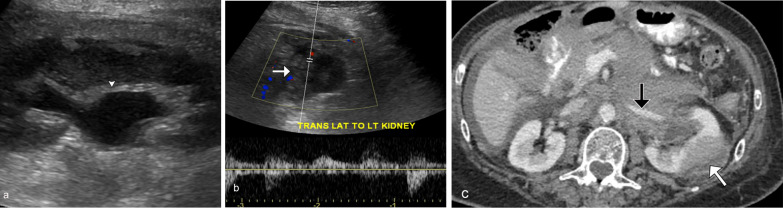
Direct extension from retroperitoneal adenopathy of renal lymphoma.* Grayscale ultrasound image (a) demonstrates mild hydronephrosis (arrowhead) of the left kidney. Spectral Doppler ultrasound (b) demonstrates a hypoechoic mass (*white arrow*) arising from the lateral left kidney with arterial waveform. Axial contrast CT (c) confirms the left renal mass (*white arrow*) with additional extensive retroperitoneal and peritoneal infiltrative disease encasing the left renal hilum. Note the maintained flow and normal caliber left renal artery (*black arrow*) despite tumor burden, characteristic of lymphoma. This patient is known to have follicular lymphoma with high grade B-cell lymphoma transformation
